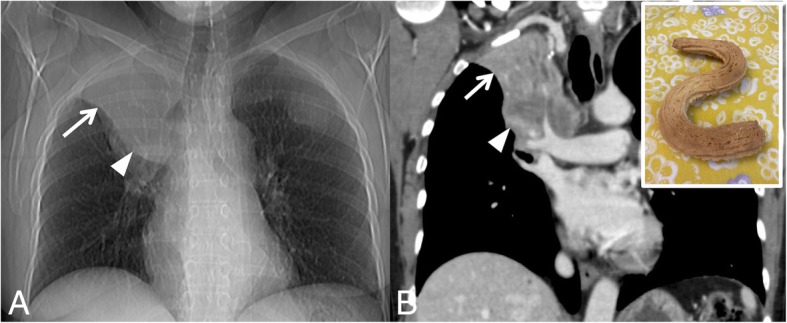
Golden S sign. The Golden S-sign reproduced in a patient affected by right pulmonary carcinoma. An “S” profile is clearly shown in a: this appearance is created by the right upper lobe atelectasis (superior and lateral part of the S profile) and by pulmonary neoplasm at the right hilum (inferior and medial part of the S profile). The same appearance is well depicted in coronal MPR CT image (b), very similar to the “S” profile of the biscuit reproduced in the embedded figure
